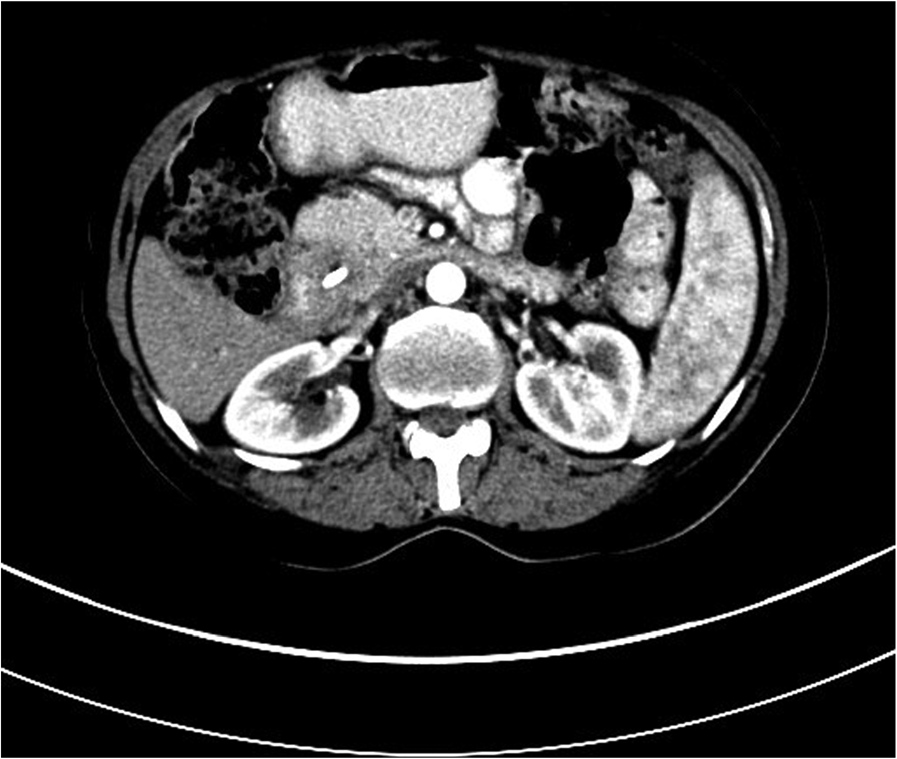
Arterial phase CT scan of a 54-year-old woman shows a high-density stent in the bile duct and a hypodense tumoral lesion in the periampullary region
